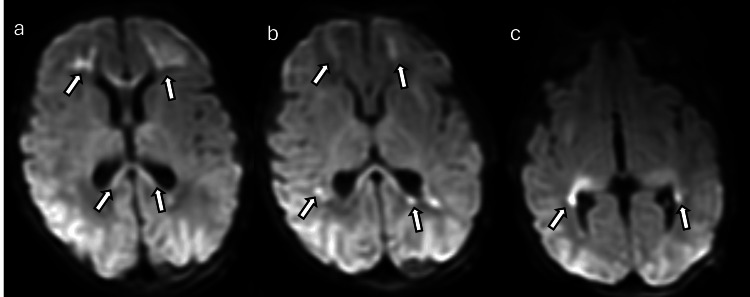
Human parechovirus meningoencephalitis in a 12-day-old female patient with sepsis and seizures (a), (b), and (c): Axial DWI images demonstrate multifocal frontal-predominant subcortical white matter and callosal involvement with associated low diffusivity (arrows) and thalamic involvement. Images obtained by the authors from the institutional PACS. All images are de-identified and used in accordance with institutional policy. DWI: diffusion-weighted imaging; PACS: picture archiving and communication system
